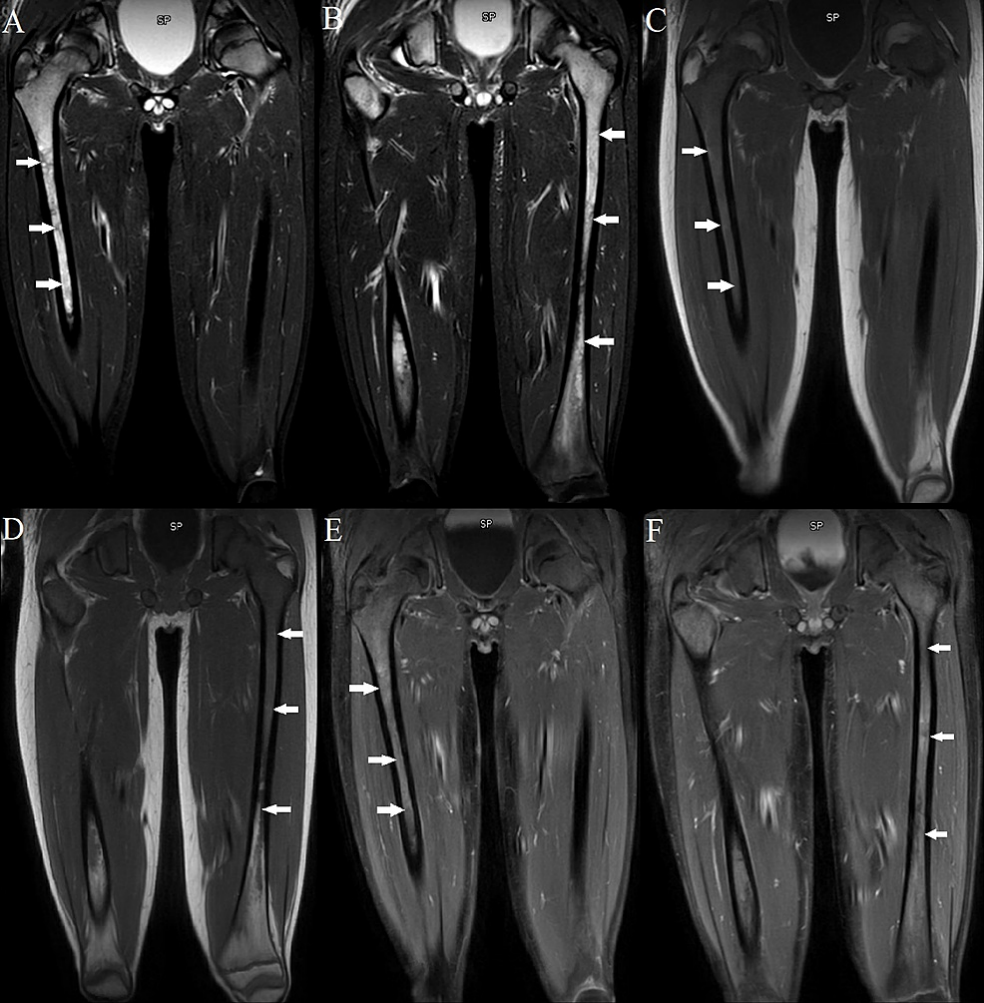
MRI of the lower extremities with and without contrast. (A, B) Coronal STIR sequence. (C, D) Coronal T1 sequence. (E, F) Coronal postcontrast T1 sequence. Note the abnormal marrow signals and heterogenous enhancement involving the bilateral femora in a pattern associated with infiltrative metastatic bone disease.
